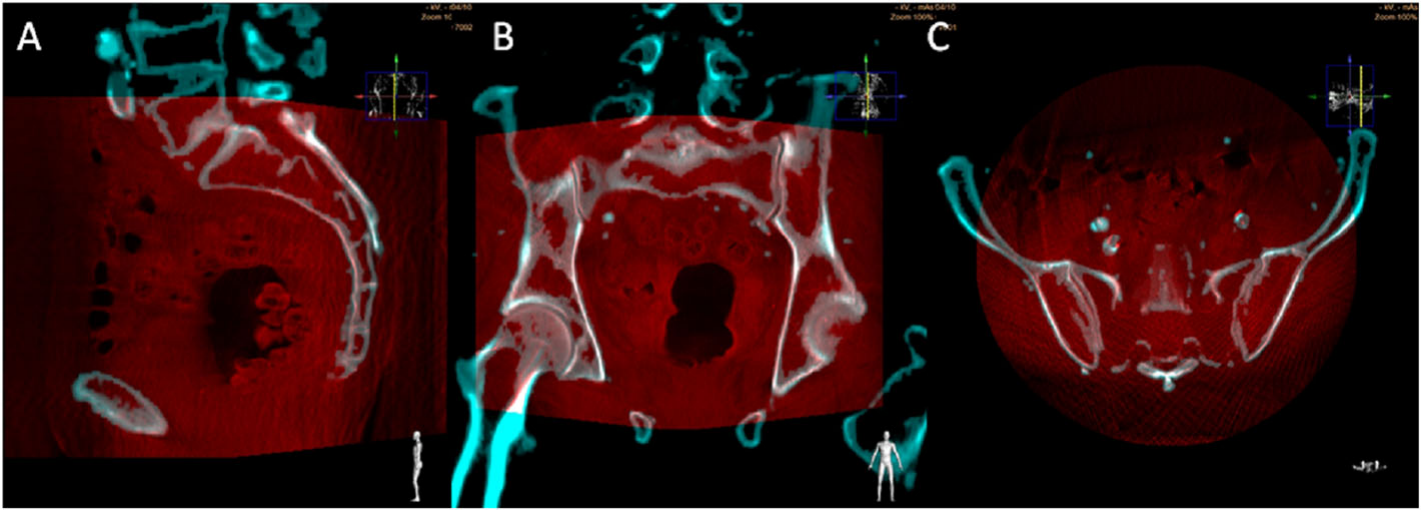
3D roadmap was created by fusing CT data with virtual vessels and the actual angiography image using the positions of the bones (a: sagittal planes, b: coronal planes, c: axial planes). Blue zone was from pre procedural CT data. And red zone was the pelvic region with 3D rotational angiography using an angiography machine and reconstructed the acquired volume data
